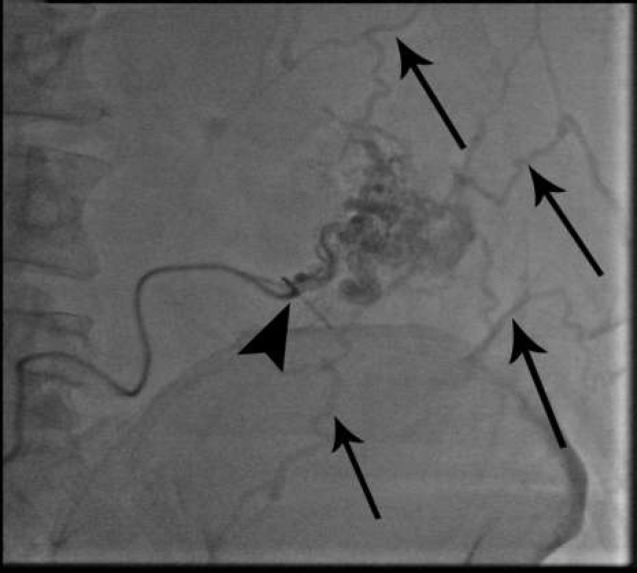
Ultrasound guide parastomal varicose vein puncture and contrast injection in inferomedial part of colostomy (mesenteric part) (arrowhead) and multiple afferent tiny subcutaneous systemic veins (arrows)
